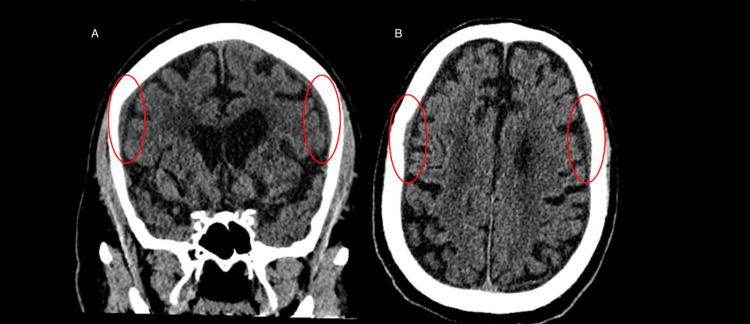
Two images showing a non-contrast head CT demonstrating bilateral subacute subdural hematomas. Panels A and B highlight areas of low-density blood accumulated in the subdural space (red circles).
